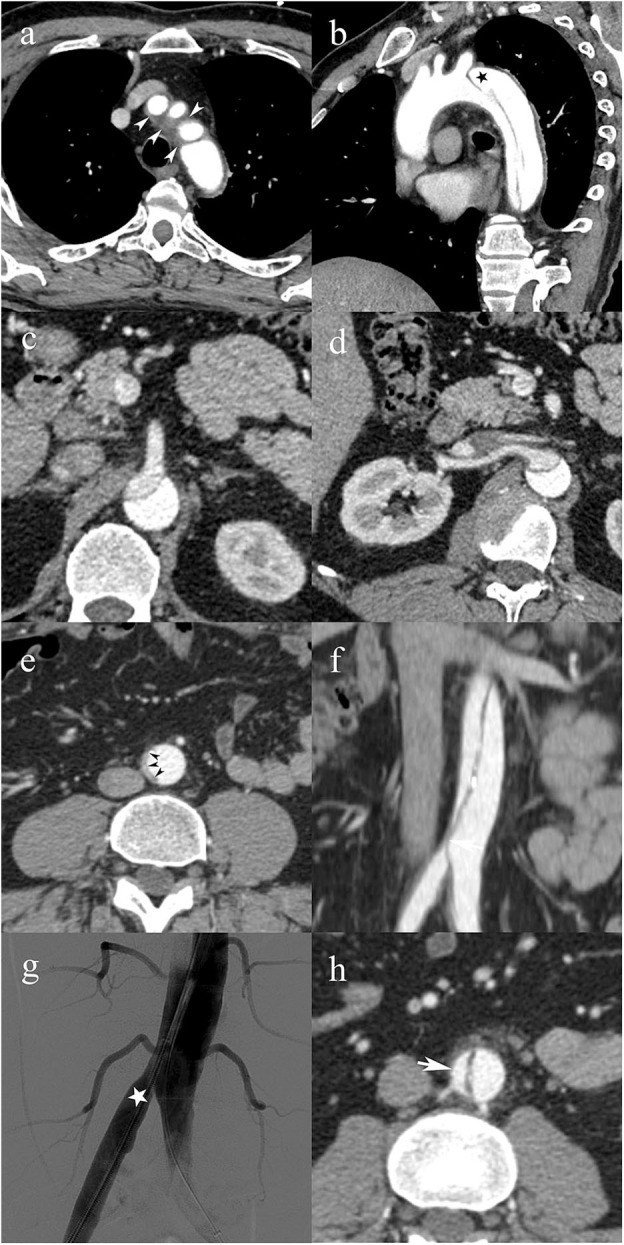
A 49-year-old male patient with type C0 AAD. Soft tissue density surrounding the origin of the supra-aortic vessels (white arrowheads) consistent with non-communicating dissection (a); patent false lumen (black star) distal to the left subclavian artery (b); superior mesenteric artery (c), and right renal artery (d) arising from the false lumen with no evidence of vascular compromise; infrarenal abdominal aorta with complete collapse of the true lumen (black arrowheads) (e); vascular compromise of the right common iliac artery due to the collapsed true lumen causing dynamic MPS of the right lower limb (f); transcatheter angiography immediately after endovascular fenestration shows restored blood flow in the right common iliac artery (g); follow-up CTA shows axial image of the infrarenal abdominal aorta with patent false lumen (h).
